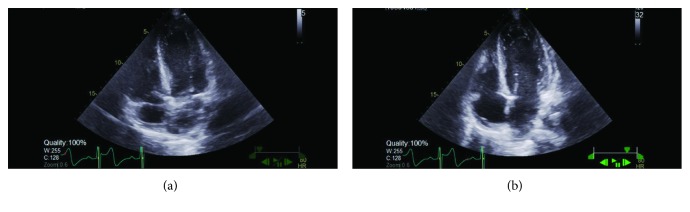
Two-dimensional transthoracic echocardiogram showing normal sized cardiac chambers. Diastole phase (b) and systole phase (a).
